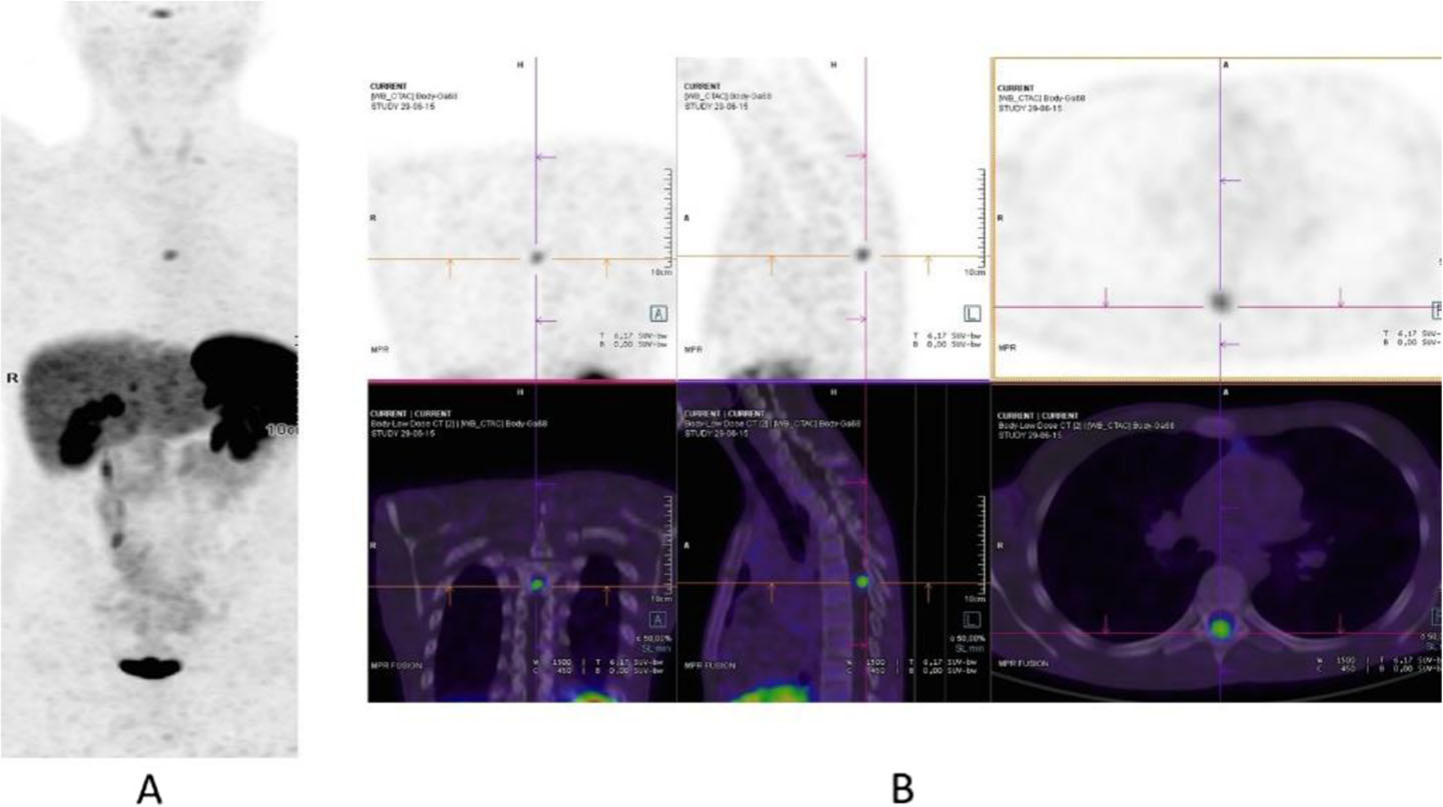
^68^Ga-DOTA peptide, suspicion of NET, specificity. *Clinical history*: 17 y.o. boy with known Von Hippel-Lindau disease. Increased level of pancreatic polypeptide. *PET/CT findings*: ^68^Ga-DOTANOC PET shows an intramedullary focus of increased uptake at the level of T8 (a MIP, b PET and fused PET/CT), corresponding to a hemangioblastoma
